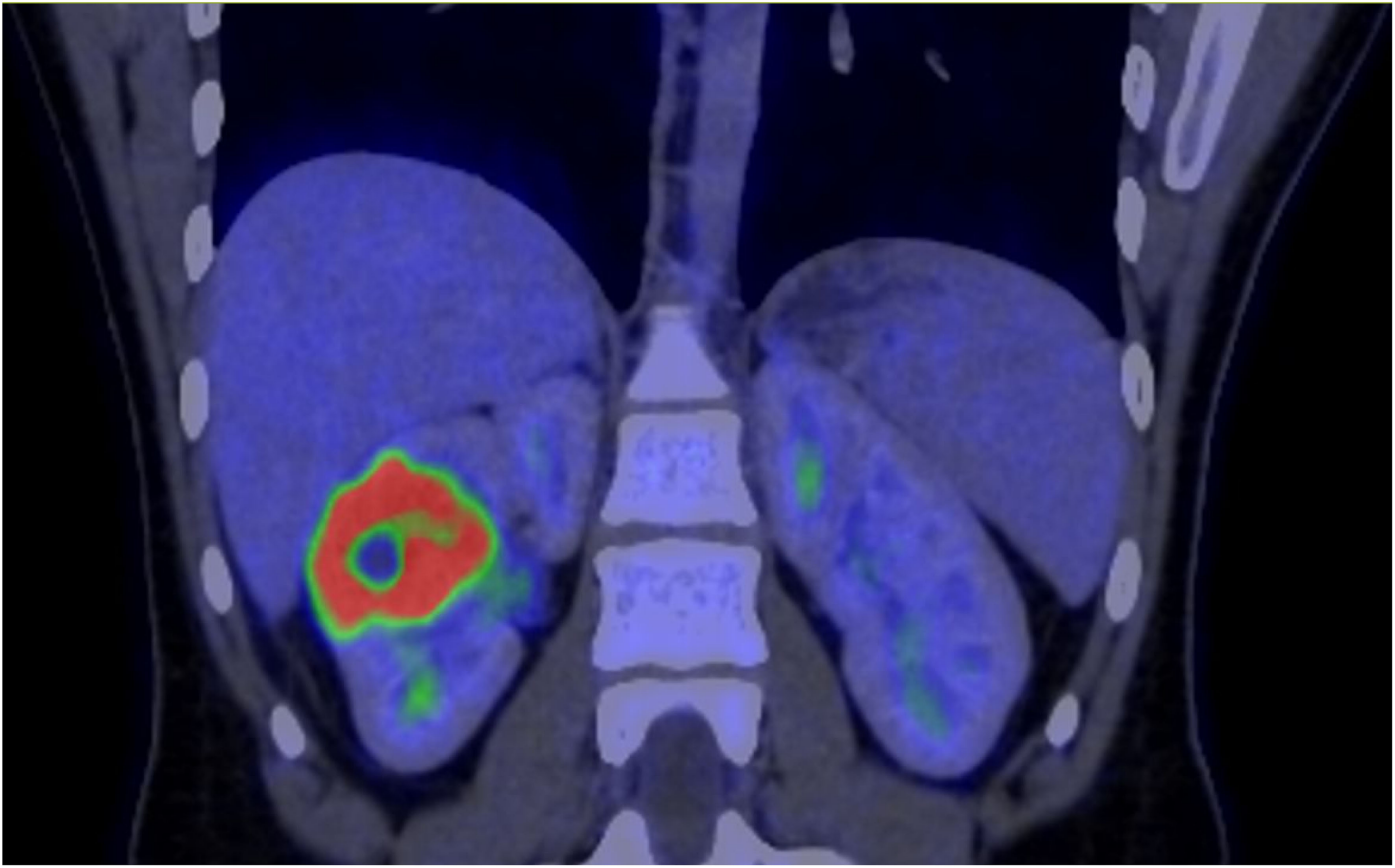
PET-CT showed evidence of a renal lesion with marked hypermetabolism and a central necrotic area in the anterolateral cortical region of the middle third of the right kidney.
